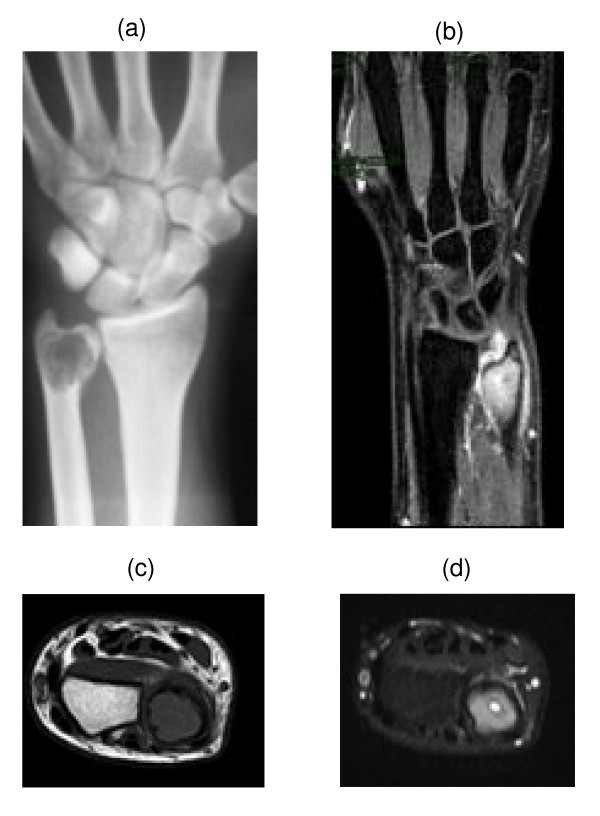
X-rays and magnetic resonance imaging with and without contrast.
